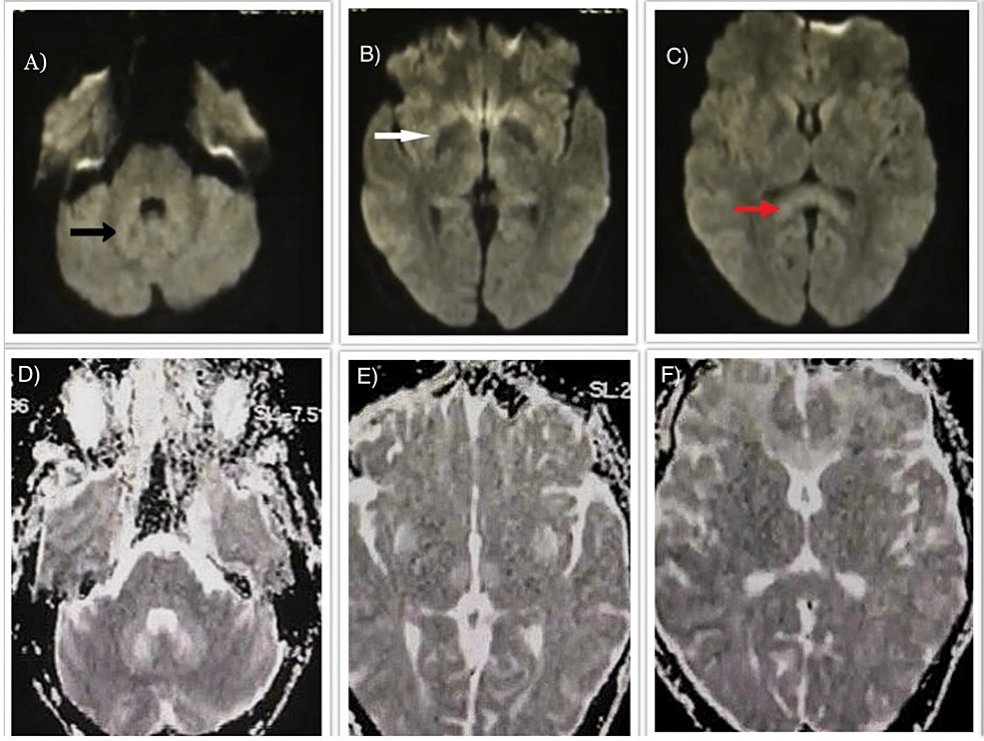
0.4 Tesla MRI and axial DWI reveal hyperintense signals in (A) dentate nuclei (black arrow), (B) basal ganglia (white arrow), and (C) splenium (red arrow). (D-F) ADC maps show high ADC areas in the dentate nuclei and basal ganglia. However, ADC values in these regions were not reduced, meaning there was no true diffusion restriction. MRI: magnetic resonance imaging; DWI: diffusion-weighted imaging; ADC: apparent diffusion coefficient
